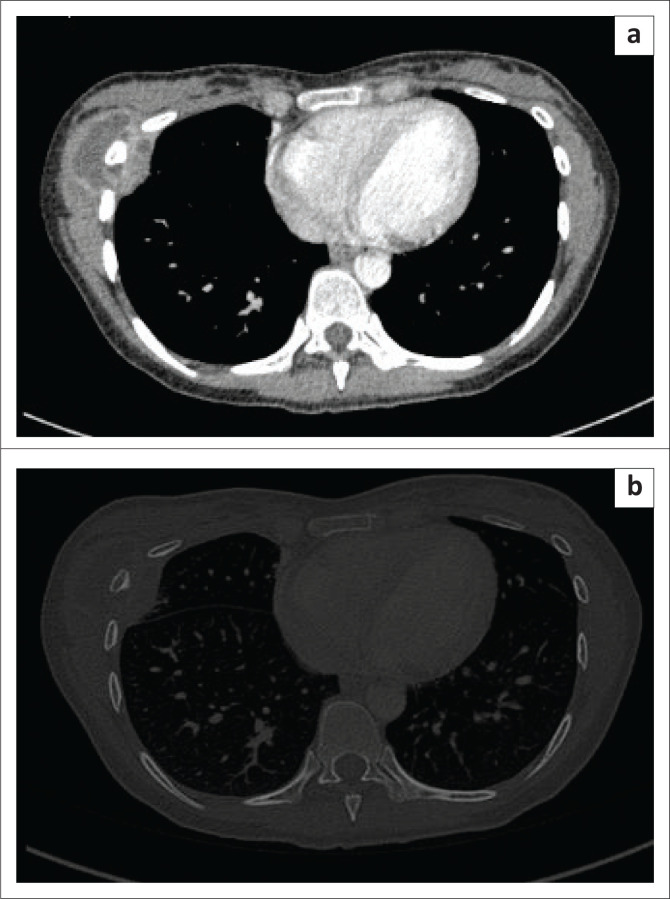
A 23-year-old female with swelling over the right chest wall. Post-contrast computed tomography axial soft tissue and bone window images (a, b) show a peripherally enhancing, hypodense soft tissue collection in the right chest wall with no obvious involvement of the underlying ribs or pleural cavity.
